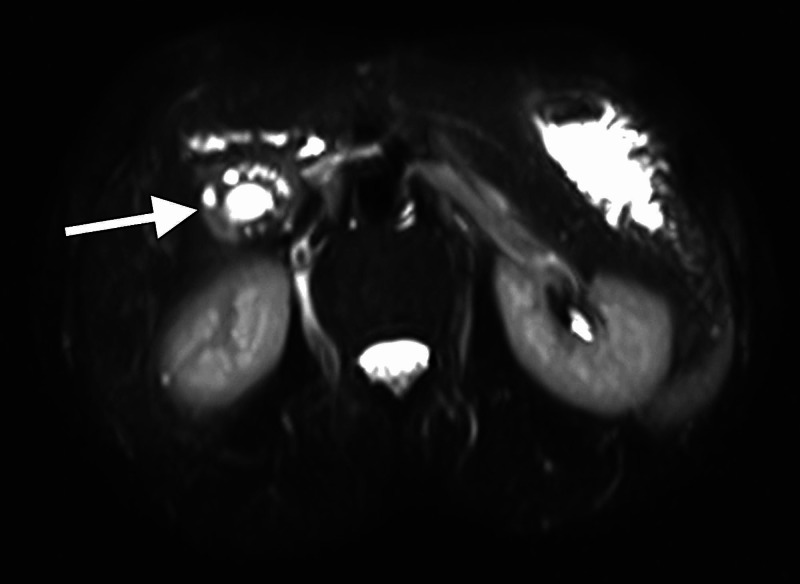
Axial T2-weighted MRI image shows mural thickening, fluid-filled intramural diverticula (arrow) representing the “pearl necklace sign”.
